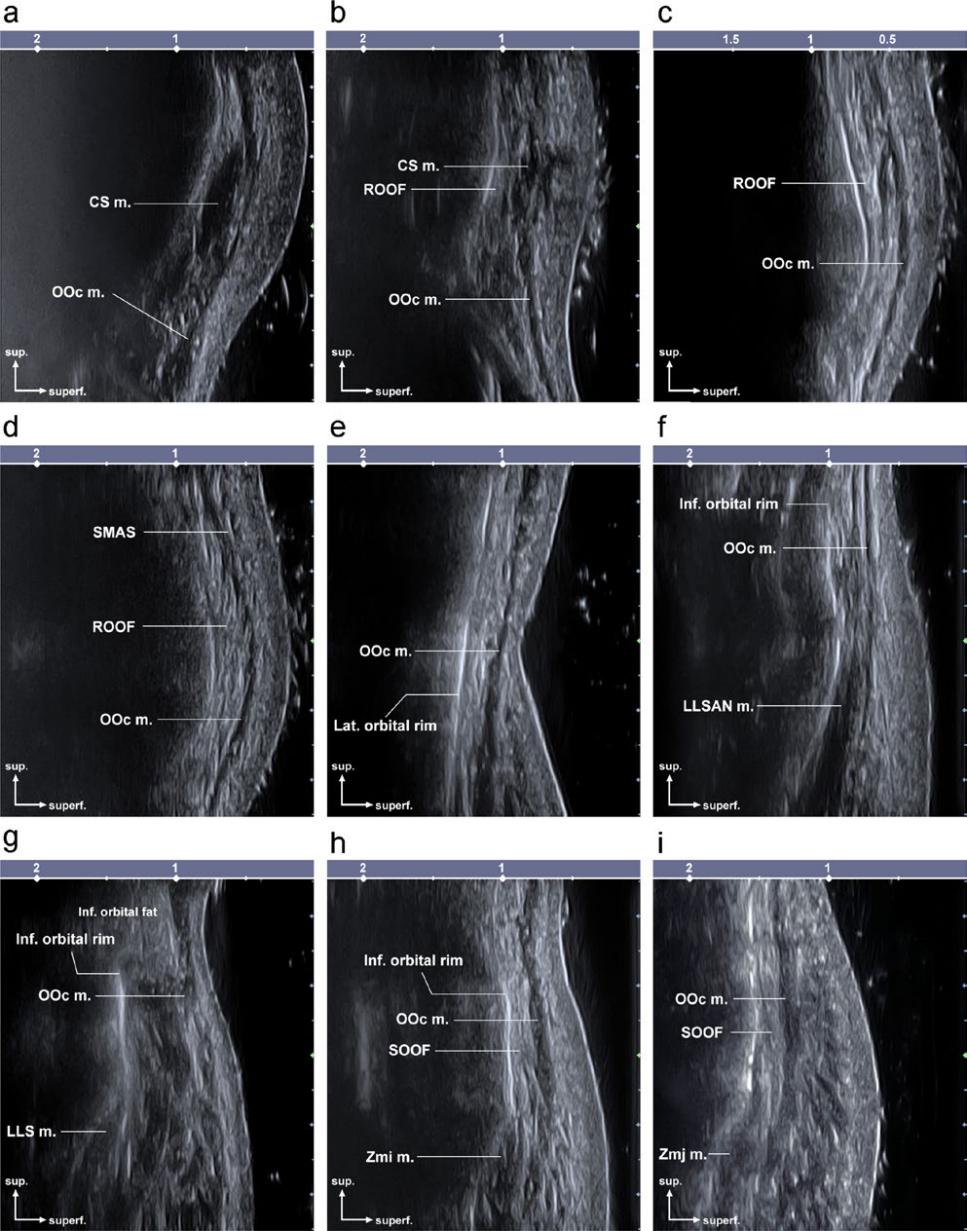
Ultrasonogram at reference points I–IX of the periorbital regions. (a), (b), (c), (d), (e), (f), (g), (h), (i); CS, corrugator supercilii muscle; LLS m., levator labii superioris muscle; LLSAN m., levator labii superioris alaeque nasi muscle; OOc m., Orbicularis Oculi muscle; ROOF, retro‐orbicularis oculi fat; SOOF, sub‐orbicularis oculi fat; Zmi m., zygomaticus minor muscle; ZMj m. zygomaticus major muscle; sup., superior; superf., superficial.
